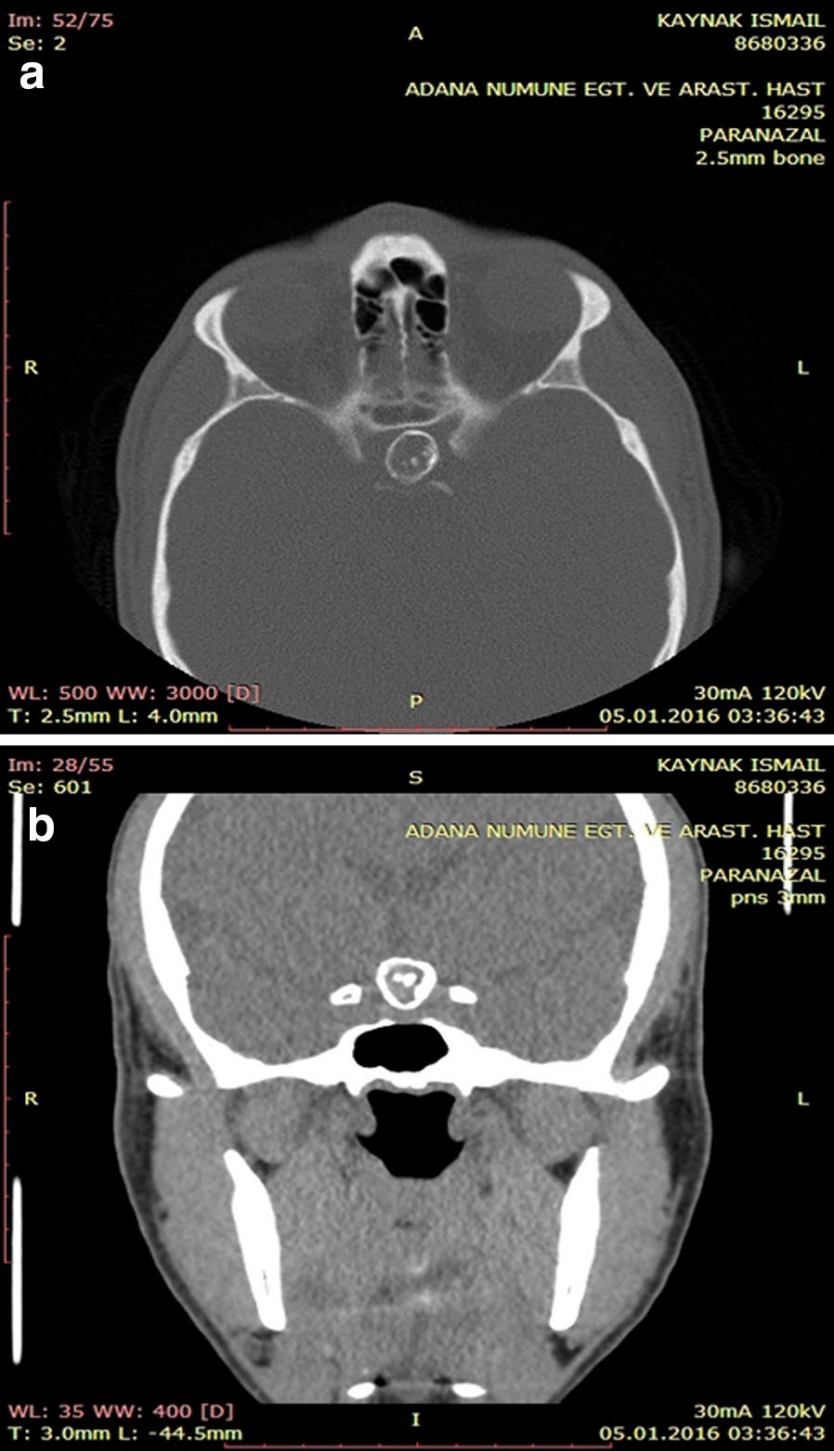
a, b The lesion was observed on the axial and coronal sections of a preoperative paranasal sinus CT of the patient
